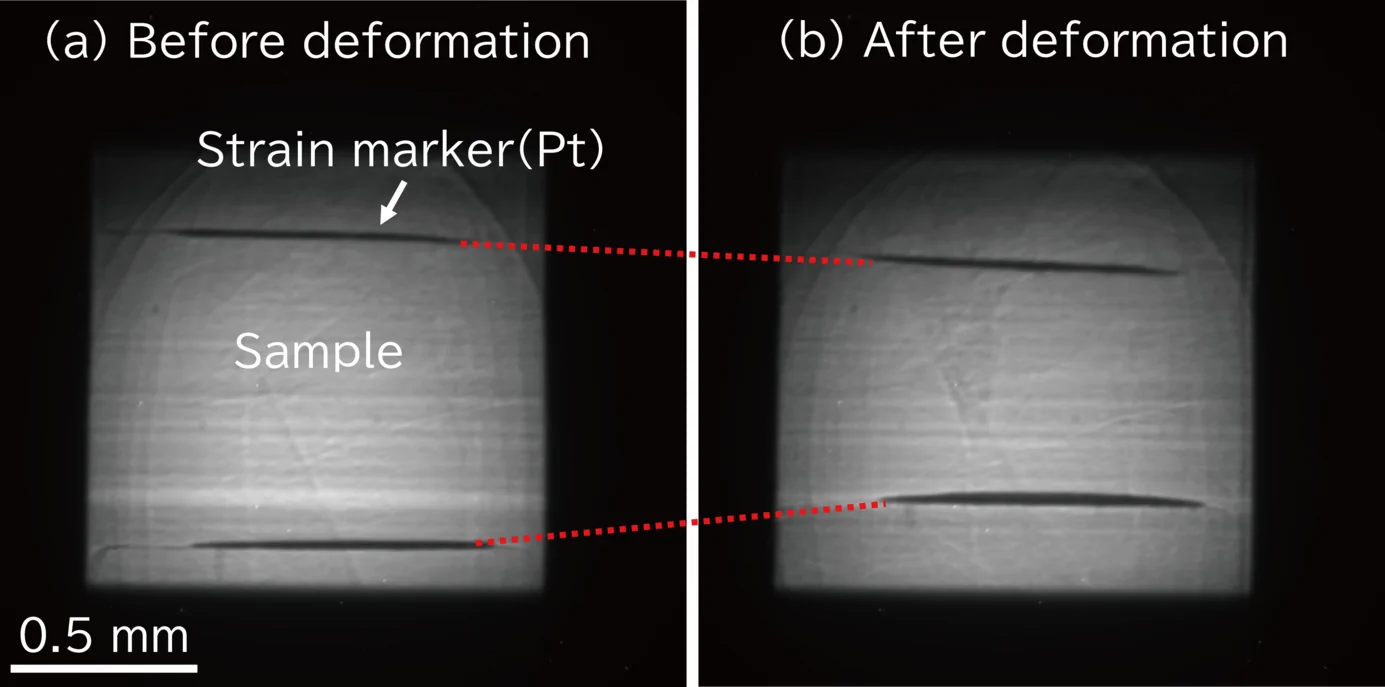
X-ray radiographs of uniaxial deformation experiments of ortho-enstatite using the MADONNA press. (*a*) Before deformation. (*b*) After deformation. Ortho-enstataite aggregate sample enclosed in Fe foil was located between Pt strain markers. After uniaxial deformation, sample length was shorter and total strain reached 28%.
